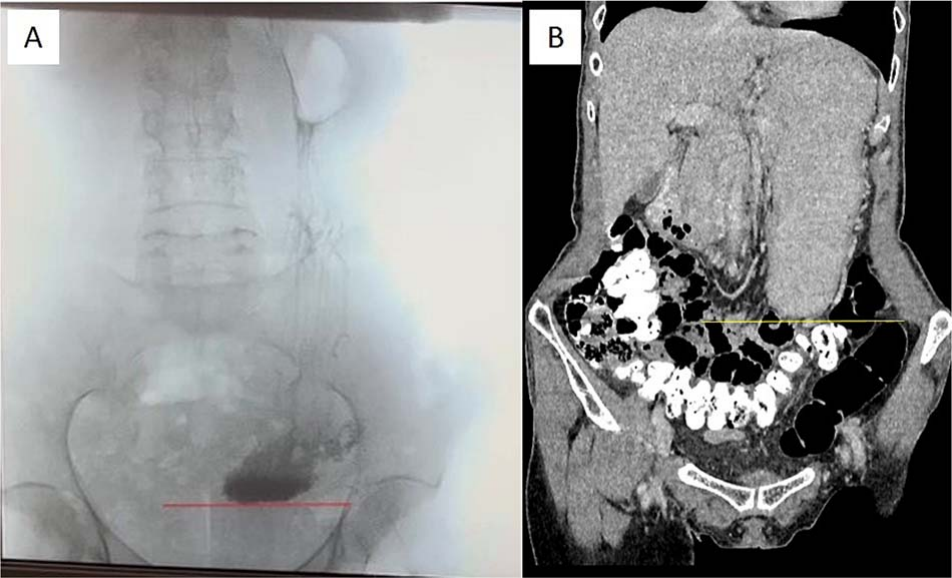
Pre-operative imaging confirming GOO. (A) X-ray of the stomach and duodenum with contrast medium. Line indicates the lower border of the stomach. (B) CT scan of the abdomen with intravenous contrast. Line demarcates the lower border of the stomach.
